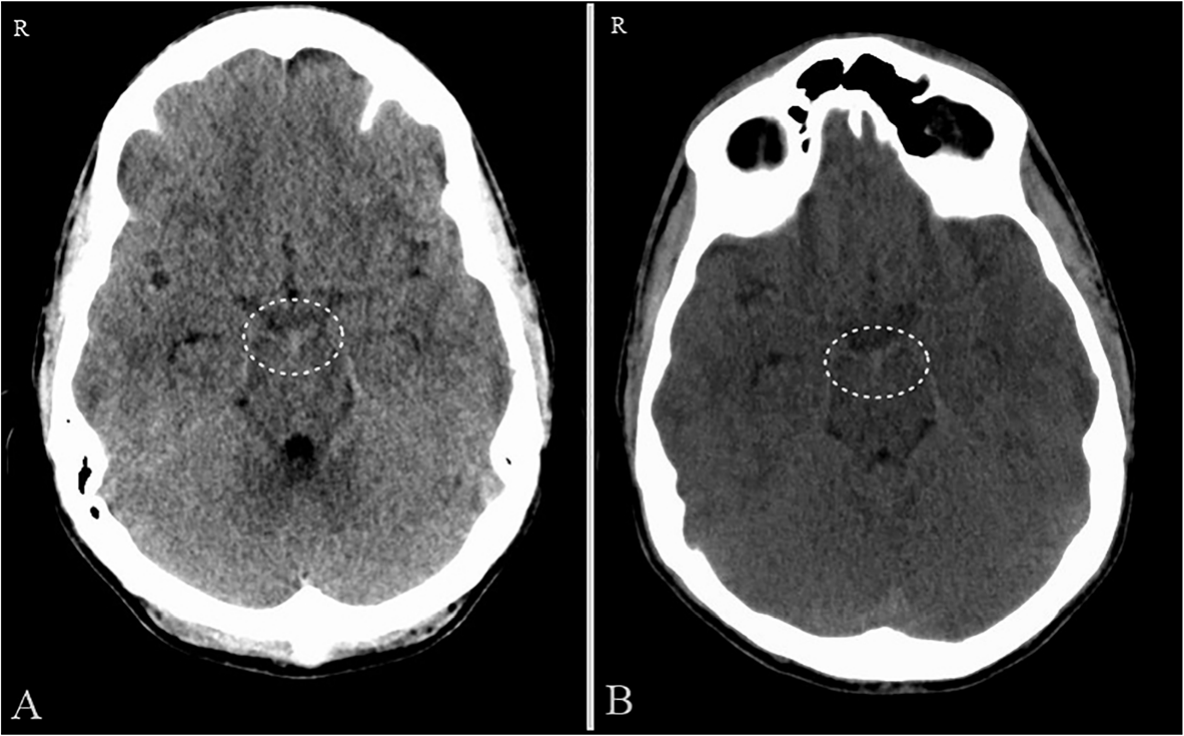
Case 1. Axial non-contrast computed tomography of the head (A and B) shows a small amount of subarachnoid blood (circle) in the interpeduncular and ambient cisterns.
